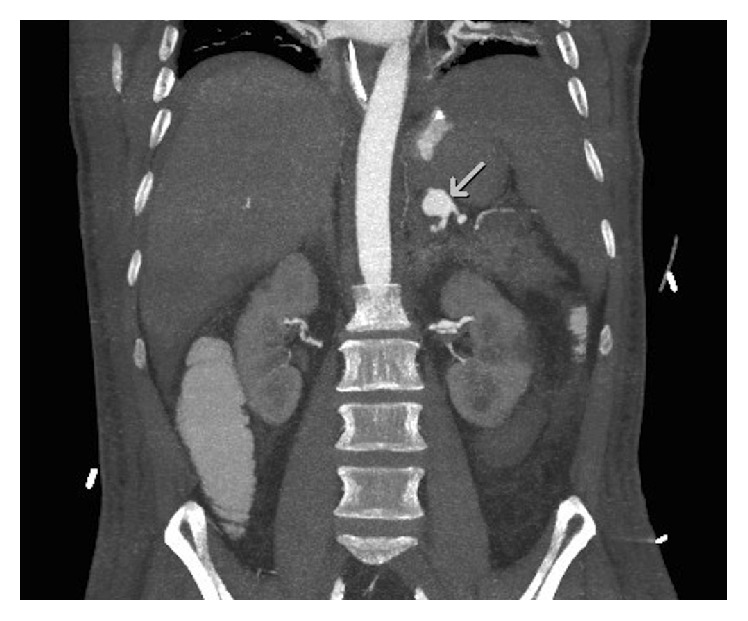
Splenic artery aneurysm (arrow) of 14 mm on abdominal computed tomographic angiography.
